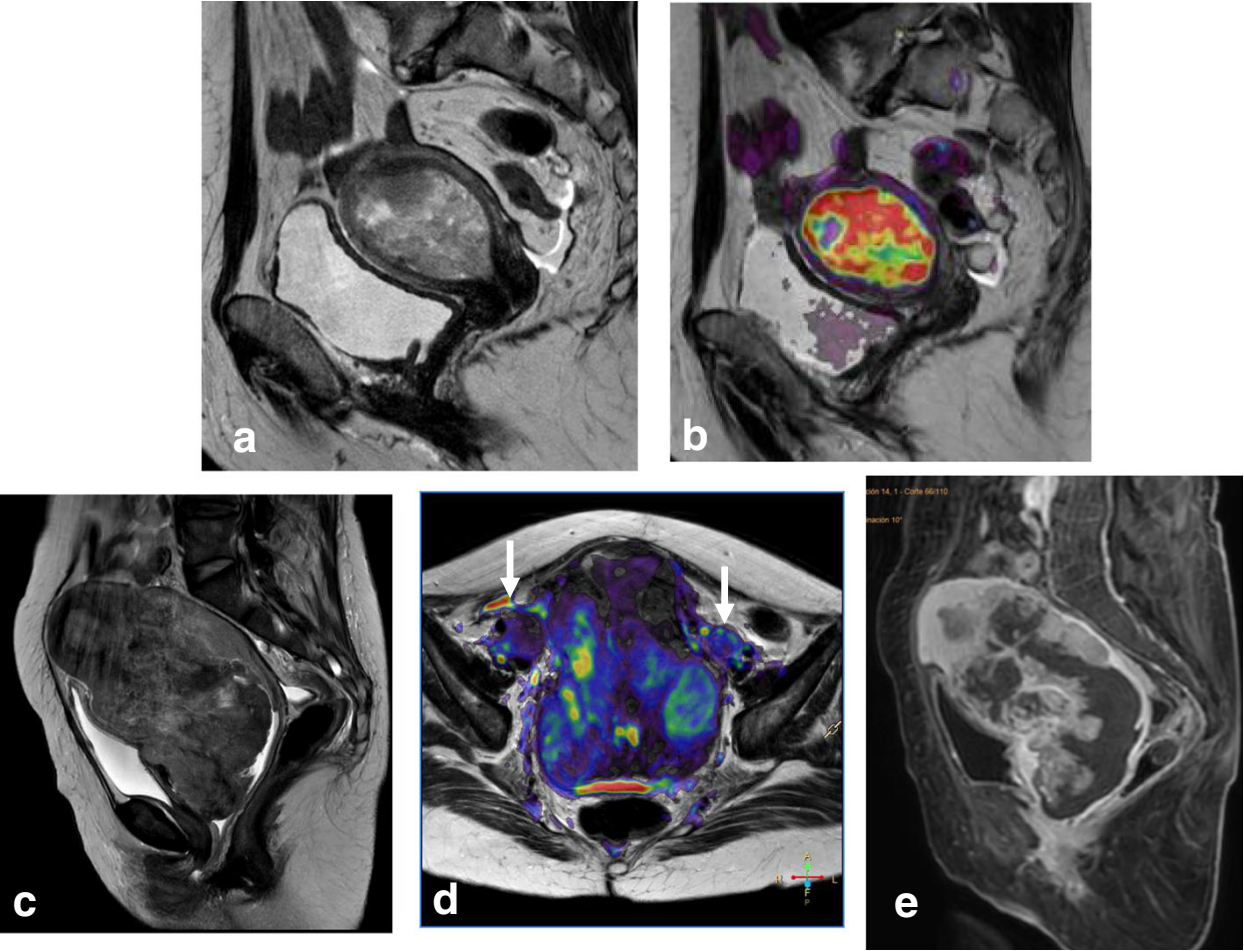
Endometrial-based tumor visualized with sagittal T2WI (a) and sagittal T2WI+DWI (b) was found to be a carcinosarcoma mimicking endometrial carcinoma. c–e In another patient, an endometrial stromal sarcoma mimicking cervical cancer is visualized by sagittal T2WI (c), axial T2WI+DWI (d), and DCE (e), revealing a cervical-centered mass with parametrial invasion and adenopathies (arrows in d)
